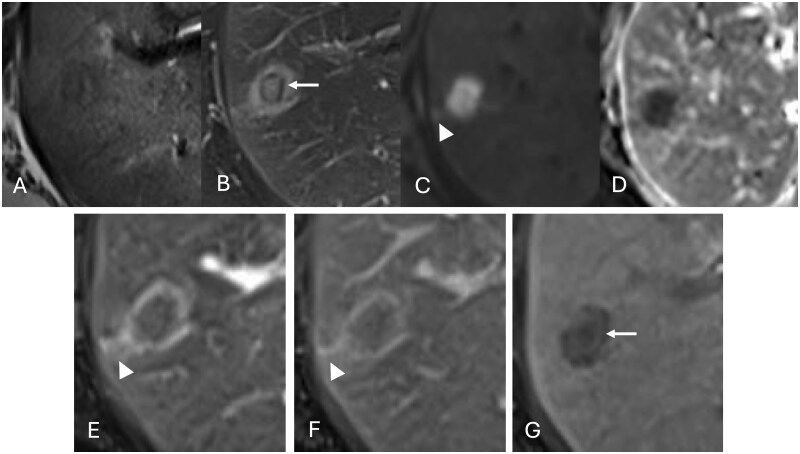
(A-C) A lesion in S5 showed hypointense on a T1-weighted image (A) and hyperintense on a fat-suppressed T2-weighted image (B) and diffusion-weighted imaging (C). The lesion contained an internal ring-like area that appeared hypointense on T2-weighted images (arow in B). (D) The ADC map of the tumor indicated an ADC value of 0.65 × 10^−3^ mm^2^/s. (E-G) On gadoxetic acid-enhanced (EOB) MRI, the lesion showed a faint enhancement in the arterial phase (E), appeared isointense relative to the surrounding liver parenchyma in the portal phase (F), and showed hypointense in the hepatobiliary phase (G). The peri-tumoral regions demonstrated early enhancement in the arterial phase (E) with persistent enhancement in the portal phase (F) and were shown to be isointense to the surrounding liver parenchyma in the hepatobiliary phase (G). There was linear hyperintensity close to the lesion on diffusion-weighted imaging (arrowhead in C) and enhancement in the arterial phase (arrowhead in E) and portal phase (arrowhead in F). The ring-like area showed a gradual, faint enhancement and hyperintensity in the hepatobiliary phase, which is distinct from the surrounding hypointense tumoral components (arrow in G).
